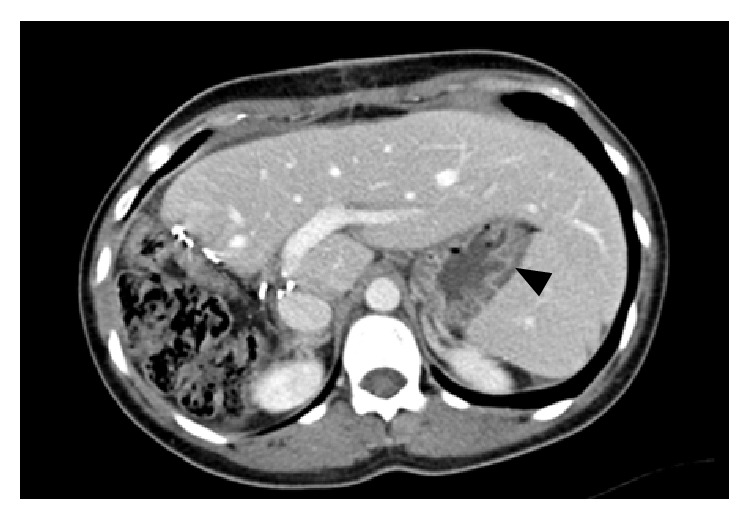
Computed tomography scan of the abdomen with intravenous contrast for patient KS, one month following open right hepatectomy. There is no evidence of adhesion formation between the stomach and cut liver surface. The stomach (black arrow) is far removed from the cut surface of the liver.
